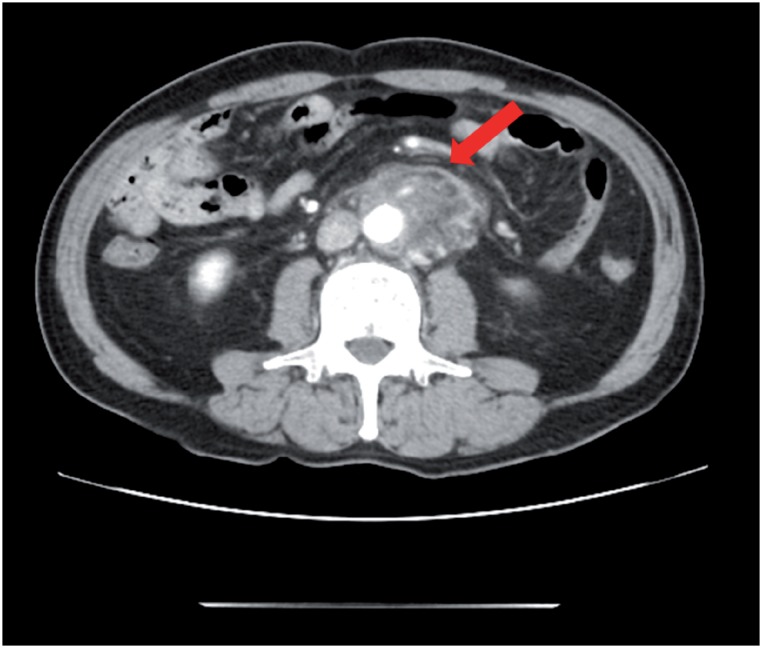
A) Enhanced computed tomography revealed an exponential increase of the low density area.
